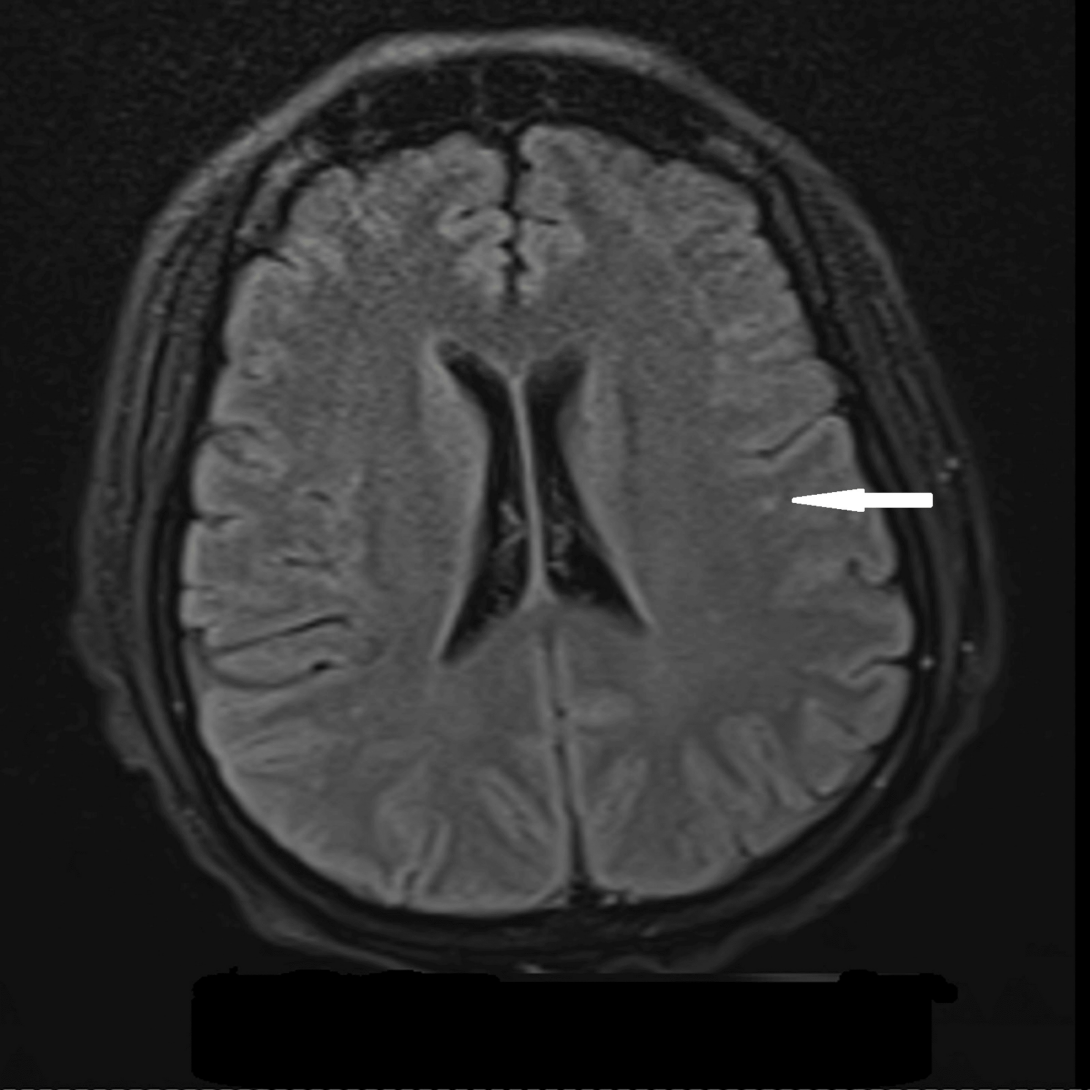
Brain MRI showing T2 hyperintense lesions marked by a white arrow on the subcortical and deep white matter areas.
